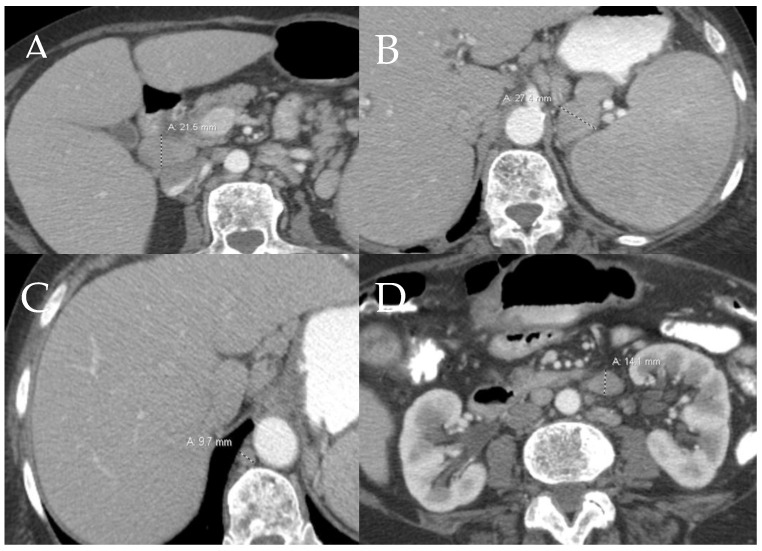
An 80-year-old female with history of aggressive systemic mastocytosis. (A–D) Axial contrast-enhanced CT abdomen shows multi-compartmental lymphadenopathy.
